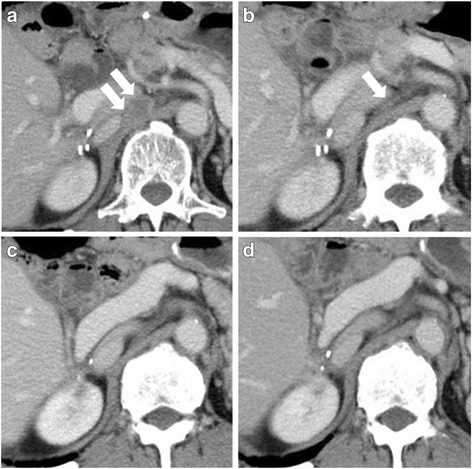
Computed tomographic images of the metastatic lymph node during the time course of treatment with regorafenib. The *arrows* indicate the metastatic lymph node.﻿ a Paracaval metastatic lymph node before the beginning of regorafenib therapy. b Metastatic lymph node decreased in size after 4 months of regorafenib therapy. c Metastatic lymph node became indistinguishable after 9 months of regorafenib treatment. d Tumor response was maintained 25 months after the beginning of regorafenib treatment
